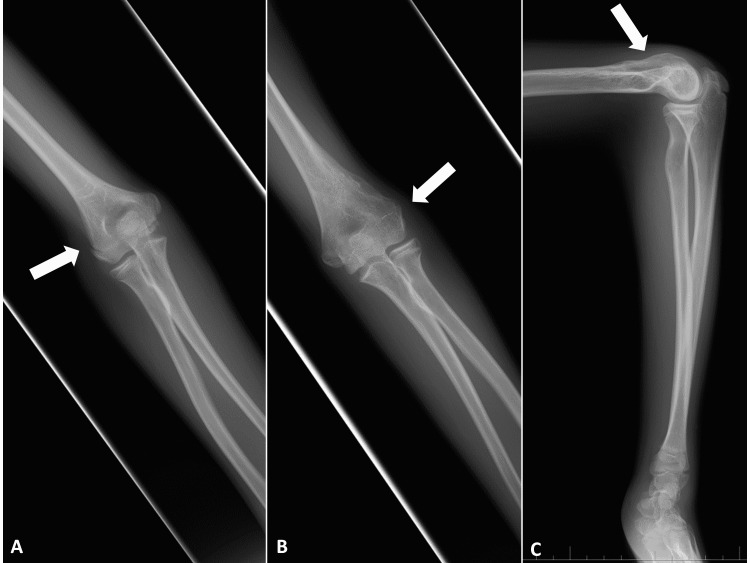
Plain radiography of the upper extremity at one and a half years postoperatively. (A) Anteroposterior view of the uninjured right elbow. (B) Anteroposterior and (C) lateral views of the left elbow. (Arrows: alignment of distal humerus).
